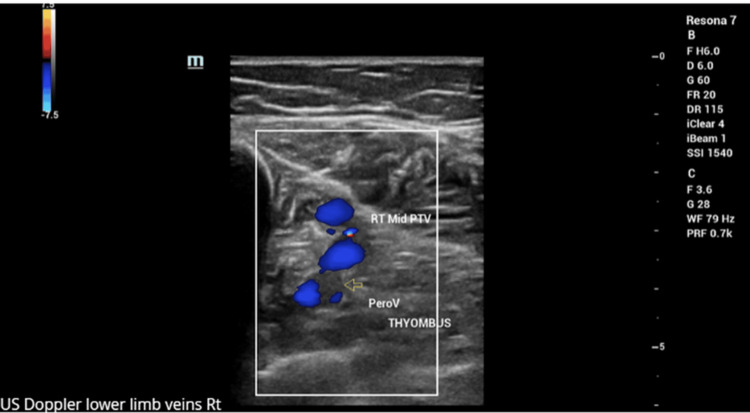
Ultrasound Doppler confirming the presence of deep-vein thrombosis.
